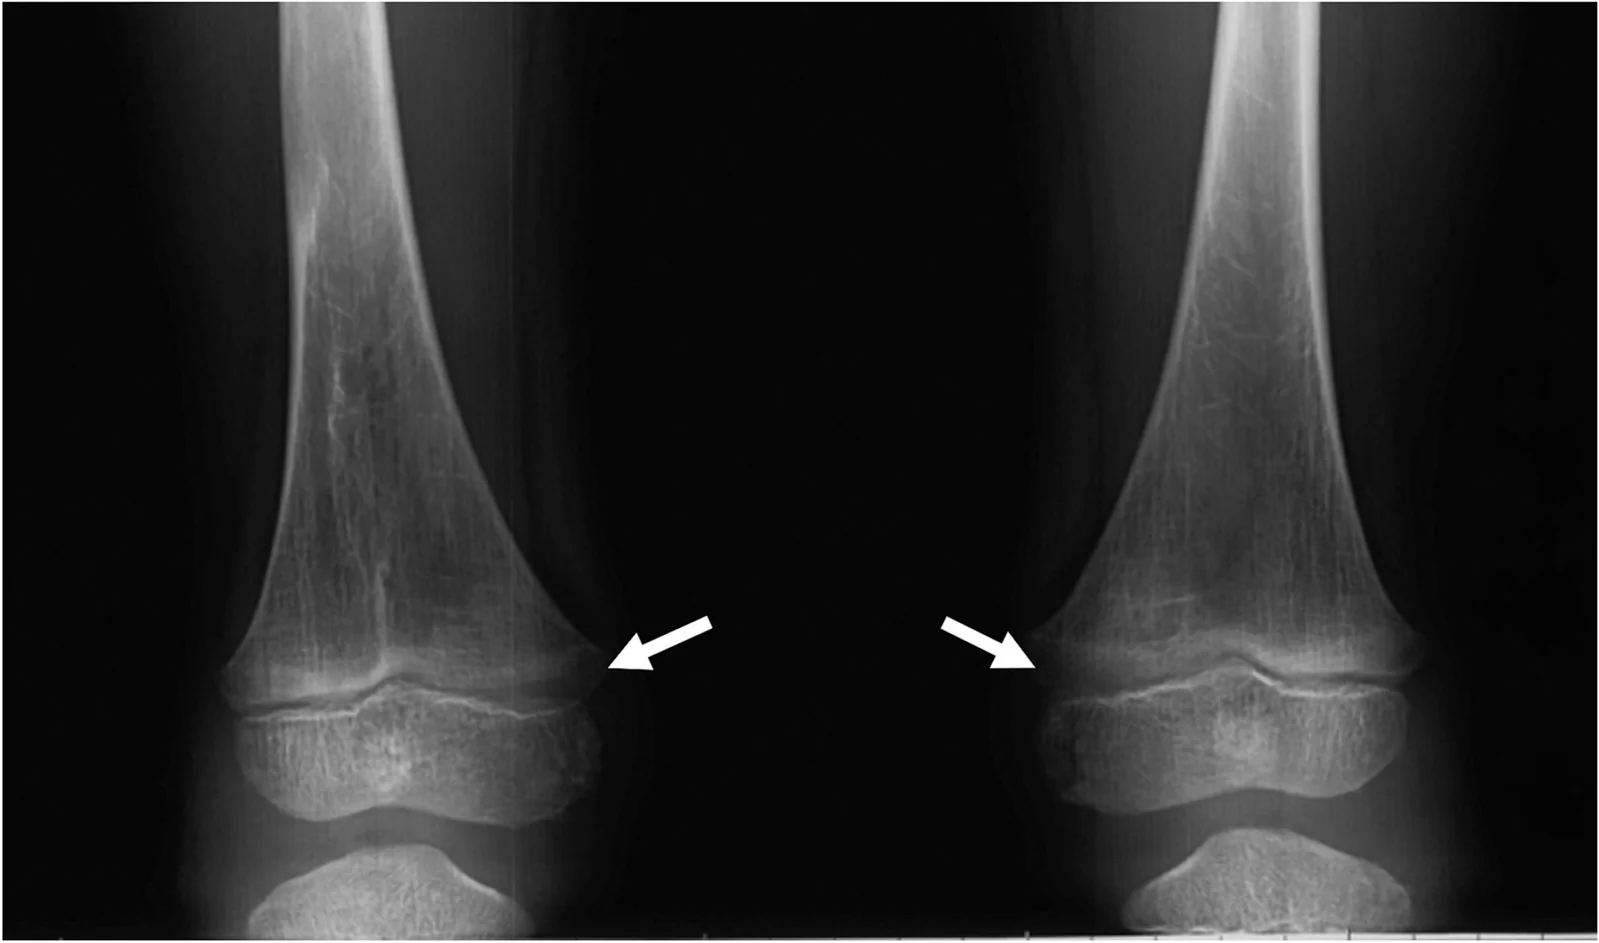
Previously unrecognized rachitic changes in Case 2 at age 5. Anteroposterior radiograph of both knees showing bilateral distal femoral metaphyseal widening, flaring, and fraying, with predominantly medial physeal widening and irregularity. Arrows indicate medial widening and irregularity of the distal femoral physes with adjacent metaphyseal fraying.
